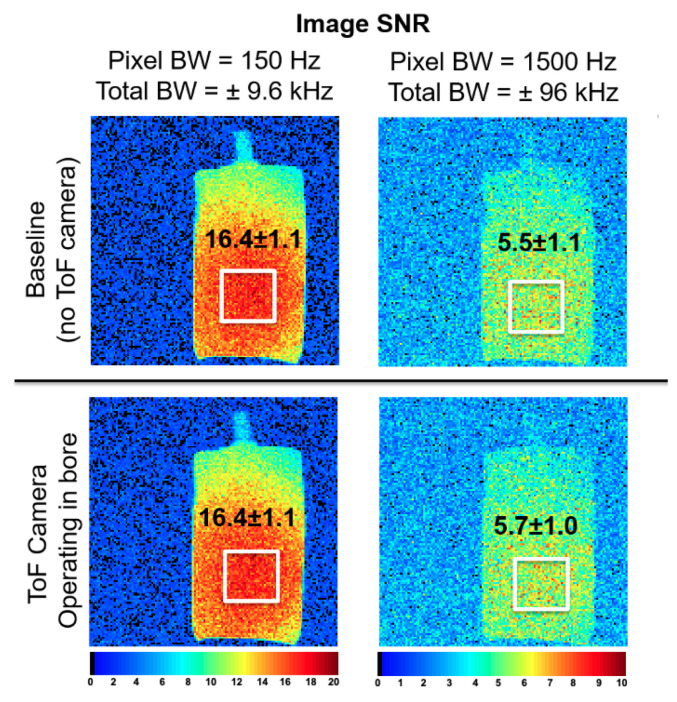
Effect of the Time-of-Flight (ToF) camera on MR image SNR. No statistically significant differences are observed between the image SNR with and without the ToF camera operational in the MR bore.
